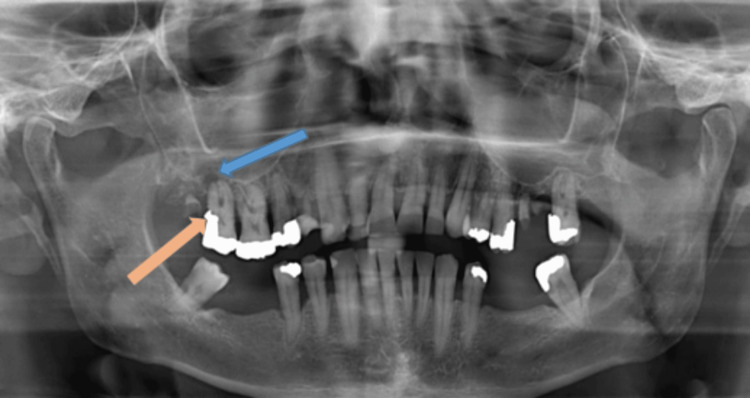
Orthopantomogram: Periapical lucency right upper second molar (blue arrow) with caries in the crown (orange arrow)
